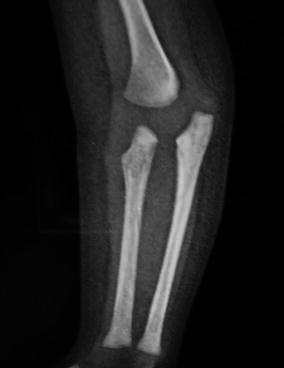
Radiograph of affected limb showing two ulnae following each other with broadened distal end and absent radius
